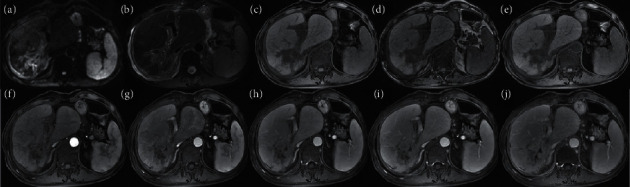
MR images in a 68-year-old HBV-negative man with iCCA confirmed by surgical resection. (a) Diffusion-weighted image; (b) T2-weighted image; (c) T1-weighted in-phase image; (d) T1-weighted out-of-phase image; and (e) T1-weighted images precontrast and in the (f) early arterial, (g) late arterial, (h) early portal venous, (i) late portal venous phases, and (j) delayed phases. The tumor measures about 60 × 88 mm and shows infiltrative appearance with delayed central enhancement.
